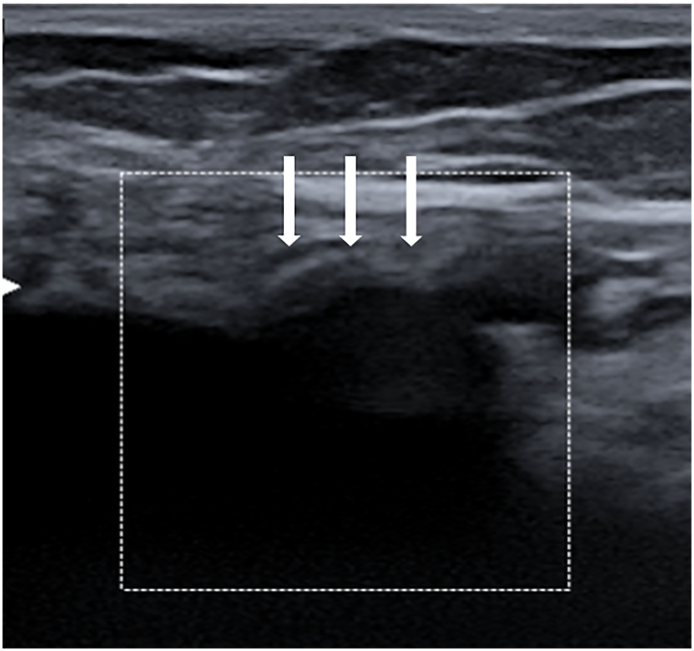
Postoperative abdominal wall sonography showing the correct position of the umbilical hernia mesh implant (white arrow).
